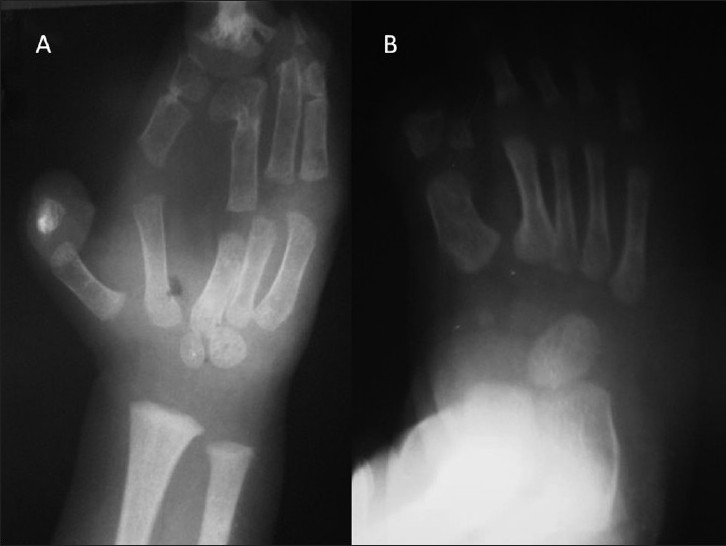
Case 1: (A) X-ray of the hand showing bony and soft tissue syndactyly with a broad, radially deviated thumb. (B) X-ray of the foot showing soft tissue syndactyly
